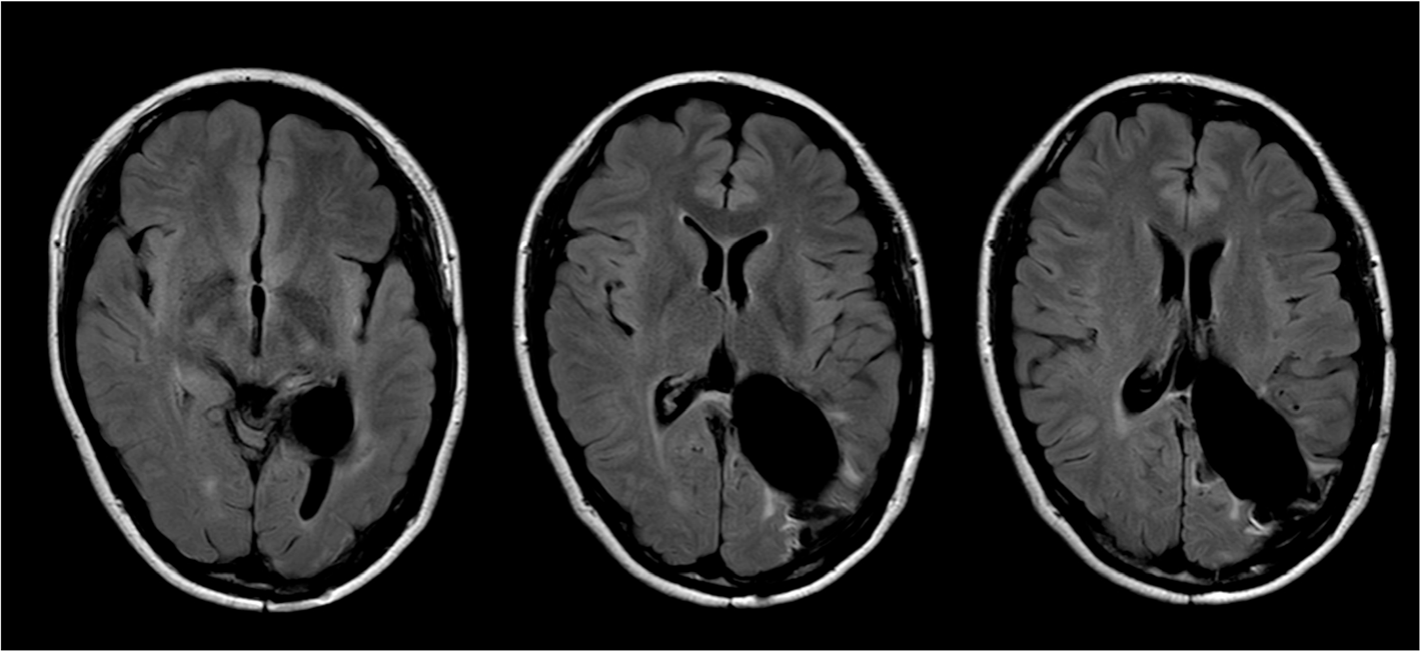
Postoperative axial fluid-attenuated inversion recovery MRI at 15 months after 2nd surgery showing total removal of the meningioma in the trigone of the left lateral ventricle
